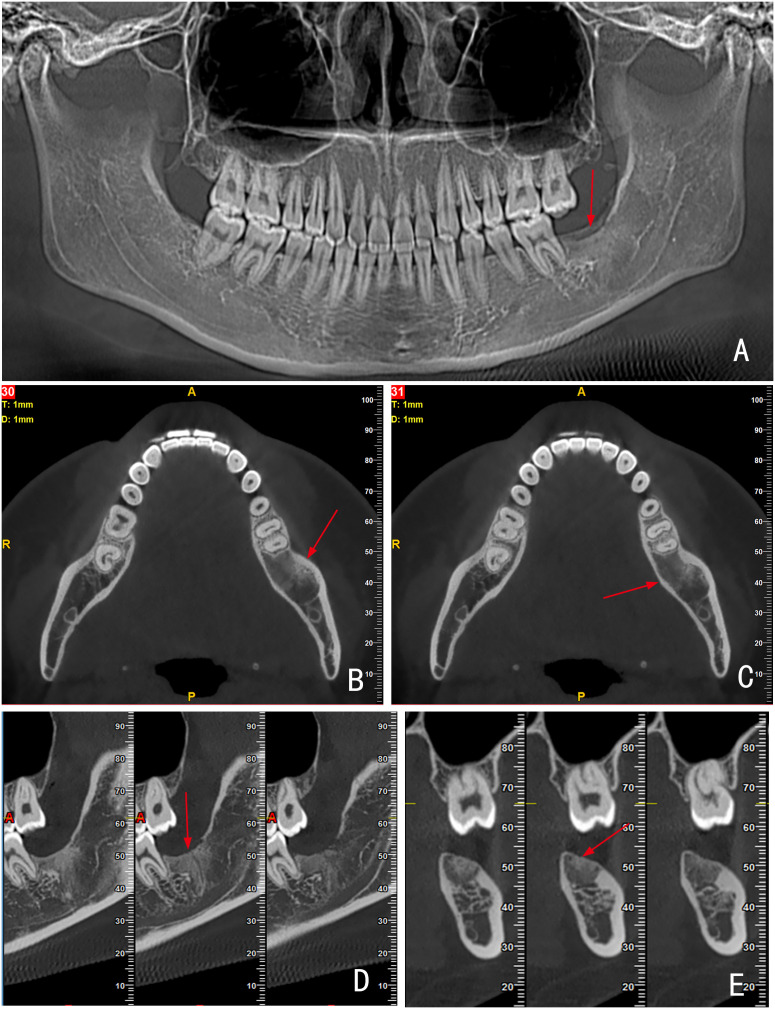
CBCT images obtained at the one-year follow-up after tumor resection. Image (A) Panoramic reconstruction; (B, C) Axial views; (D) Sagittal view; (E) Coronal view. All images demonstrate complete bone remodeling at the surgical site (red arrows).
